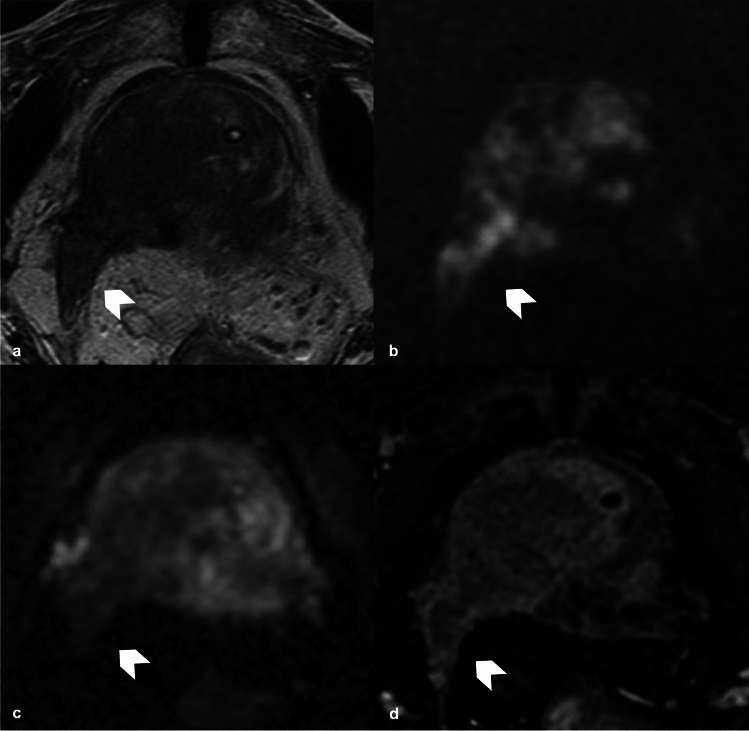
a T2WI (axial plane) shows an alteration on the right mid-base peripheral and transitional zone (white arrow head); b, c DWI (b = 2000) and ADC map show a focal and marked hyperintensity on high b value and hypointensity on ADC map on the right mid-base zone (white arrow head); d DCE shows early and focal enhancement of the lesion. All the sequences demonstrate the infiltration of the right seminal vesicle. T2WI, T2 weighted imaging; DWI, Diffusion Weighted Imaging; ADC map, Apparent Diffusion Coefficient; DCE, dynamic contrast enhancement
